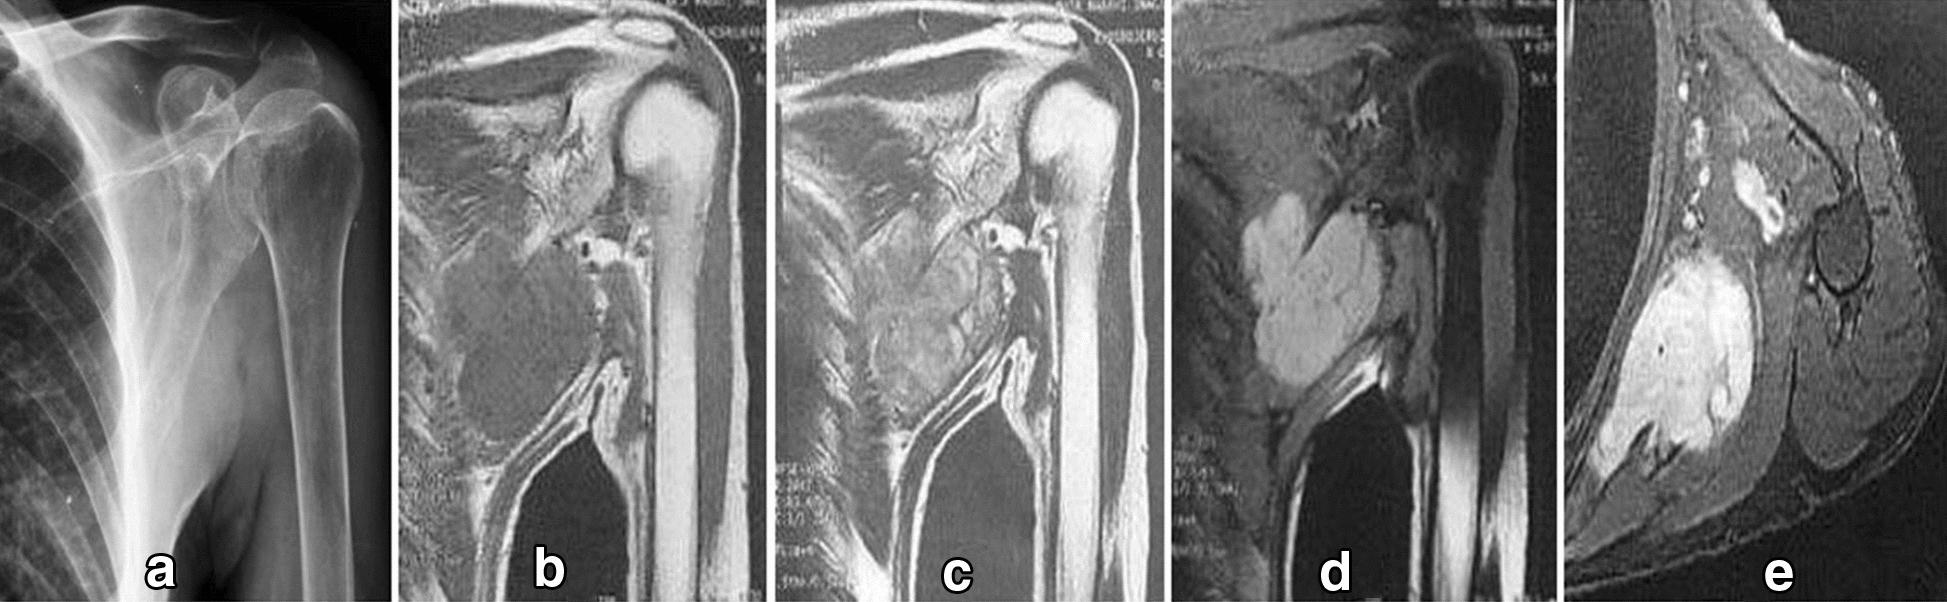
Diagnostic imaging. a Radiograph shows a soft tissue lesion affecting chest wall in the infra-axillary region. b–e, MRI shows a lobulated mass iso-signal in T1W and heterogeneous to high signal in T2W and high signal in fat-saturated sequences with strong post-contrast enhancement in the infra-axillary intermuscular fat plane. The tumor had close contact with adjacent vessels
